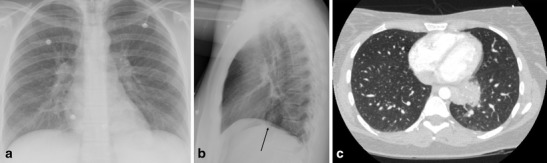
A 20-year-old old female presented with retrosternal chest pain, fever, and elevated white blood counts. (a-b) PA and lateral chest radiograph. Initially the plain radiograph was read as without abnormalities. (c) Axial chest CTA, performed under suspicion of a pulmonary embolism shows a consolidation in the left lower lobe, which can be seen as a subtle abnormality on the lateral chest x-ray in (b) (arrow)
